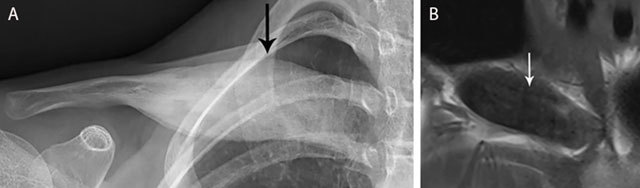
CRMO of the medial clavicle. Plain radiograph (a) shows an expansile lesion (black arrow) of the medial clavicle with extensive bone sclerosis and a solid periosteal reaction. The lesion is of hypo-intense signal on coronal T1–WI (b). Note osseous expansion of the lesion beyond the original cortex of the clavicle (white arrow).
